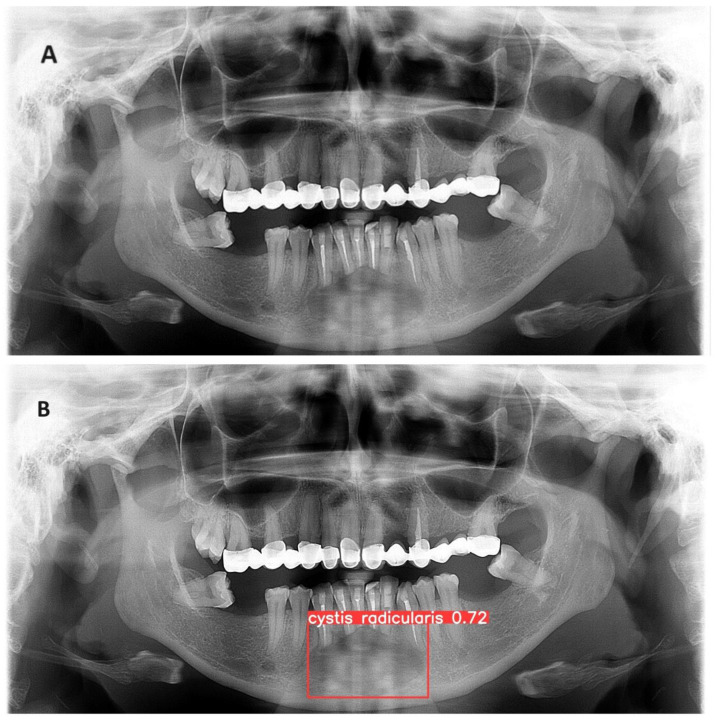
(A) Panoramic radiograph of radicular cyst in the lower jaw with surgically removed roots (B) Developed model detection of a radicular with precision of 72%.
